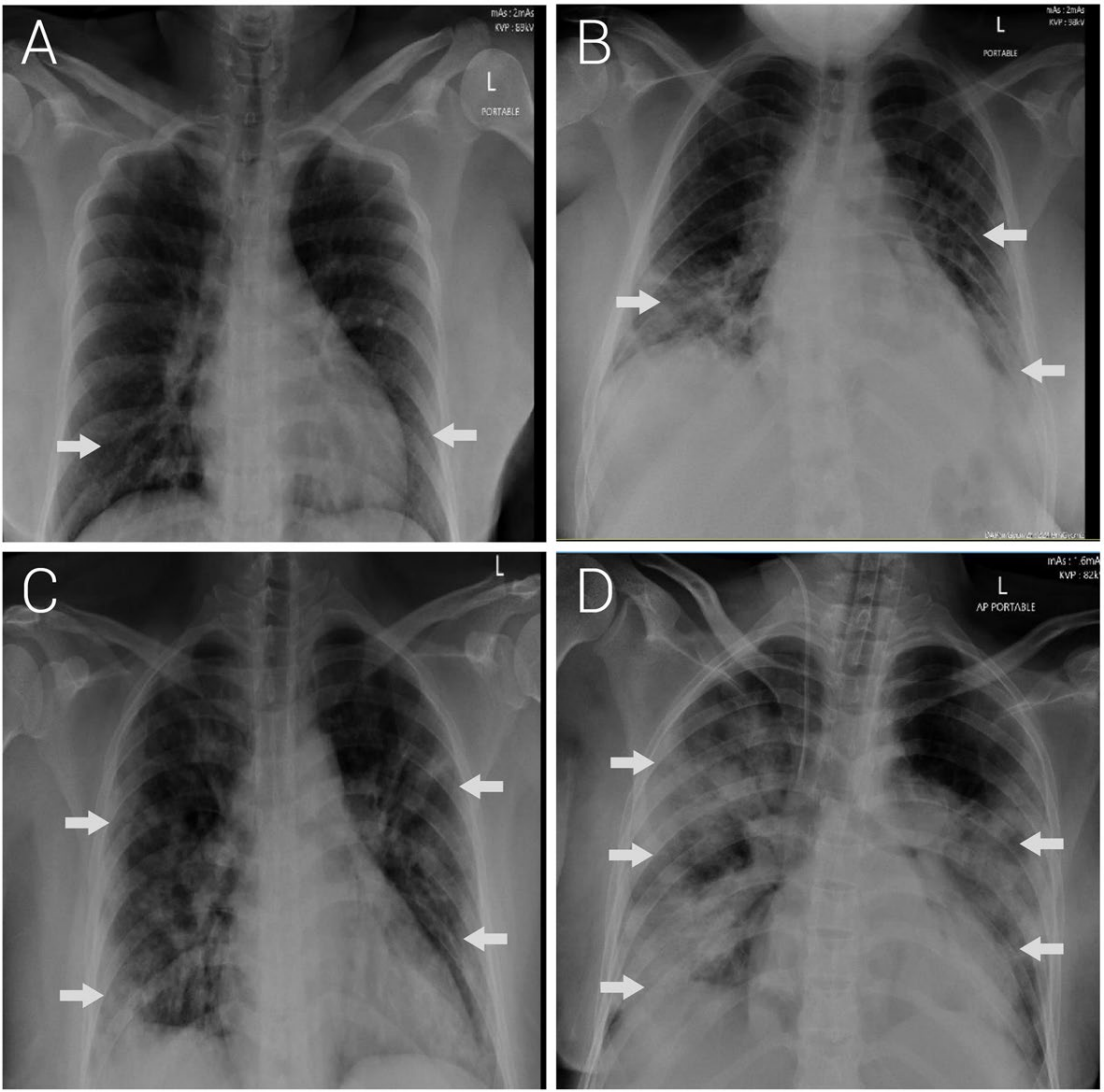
Anteroposterior radiographs depicting Covid-19 pneumonia images of pregnant women with confirmed SARS-CoV-2 infection. Images were obtained at Latifa Women and Children Hospital, Dubai, from March to June 2020. A Bilateral ground glass opacities involving two lung zones with associated linear opacities seen in the periphery of the right lower zone. B Ill-defined patchy ground glass opacities over three lung zones. C Bilateral multifocal peripheral lung ground glass opacity and consolidation involving four lung zones. D Bilateral peripheral dense consolidation with loss of lung markings in five lung zones
